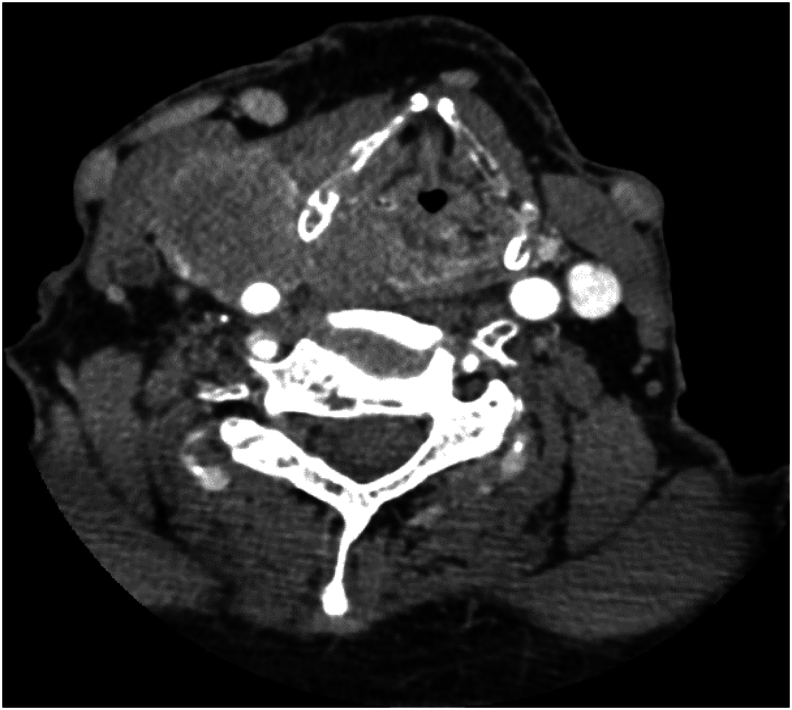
Axial CT neck with contrast showing large mucosal-bases enhancing lesion in the right hypopharynx involving the thyroid cartilage, with through-and-through local extension.
